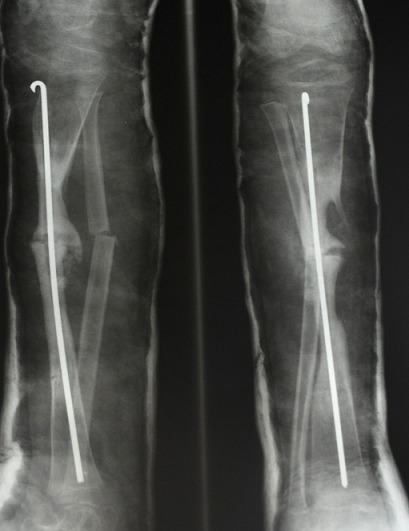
Lateral and AP radiograph of the right leg showing correction of a non-union fracture of the tibia with a rush rod
